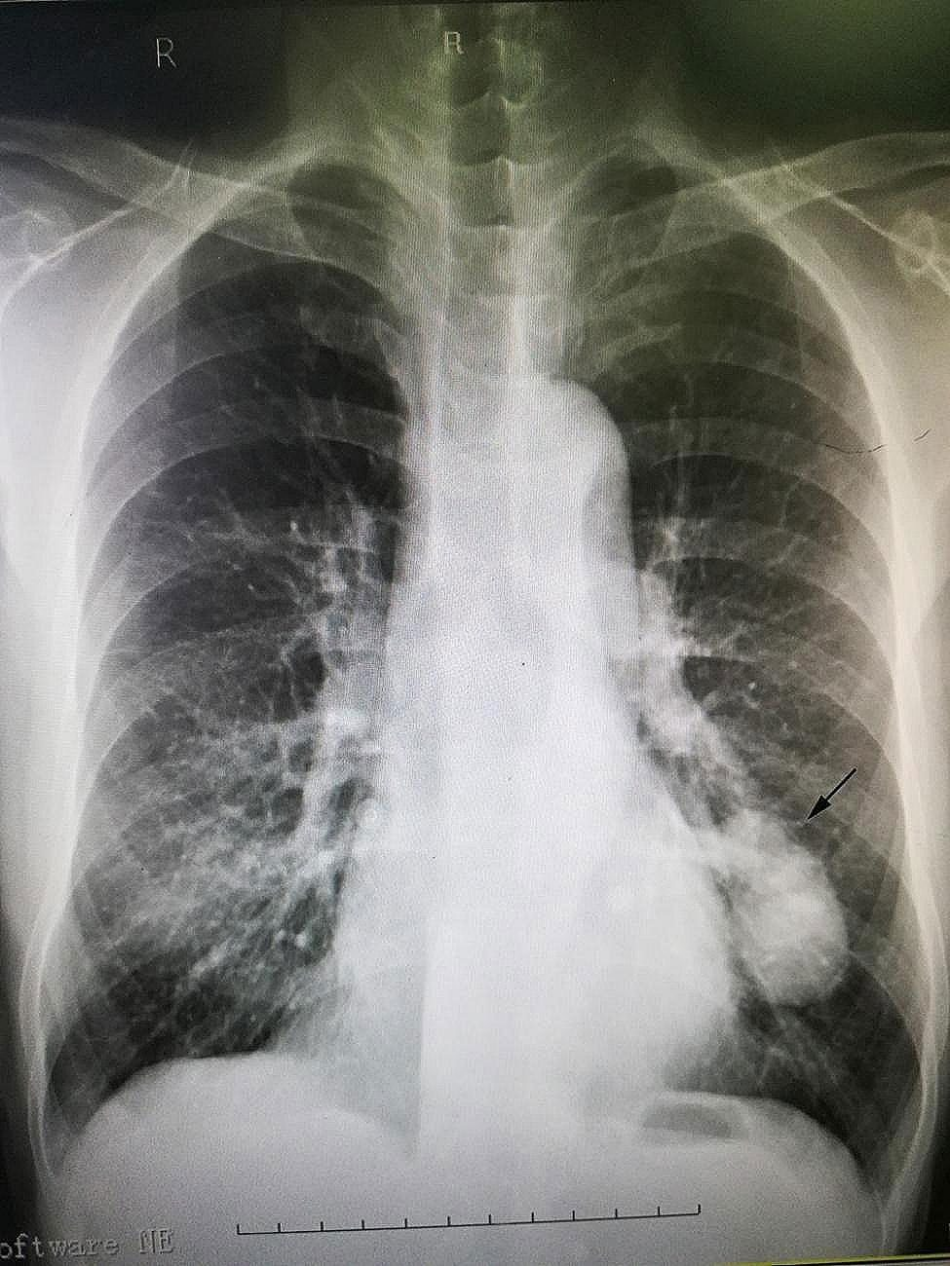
X-ray showing a mass shadow in the lower lobe of the left lung
